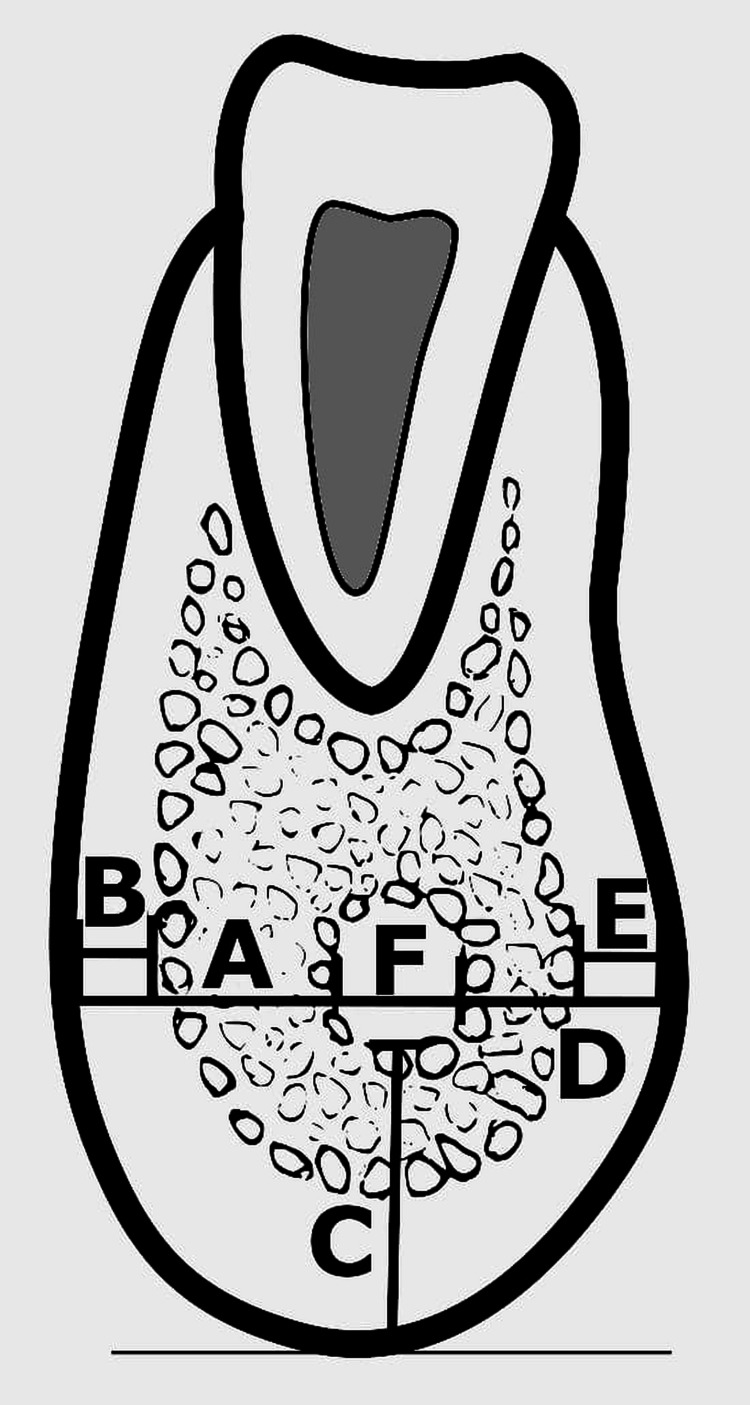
Coronal section of mandible indicating horizontal and vertical measurements in CBCT A is the distance from the canal to the buccal cortex of the mandible. B is the thickness of the buccal cortical plate. C is the distance from the canal to the lower border of the mandible. D is the distance from the canal to the lingual cortex of the mandible. E is the thickness of the lingual cortical plate. F is the diameter of the canal. CBCT: cone-beam computed tomography
